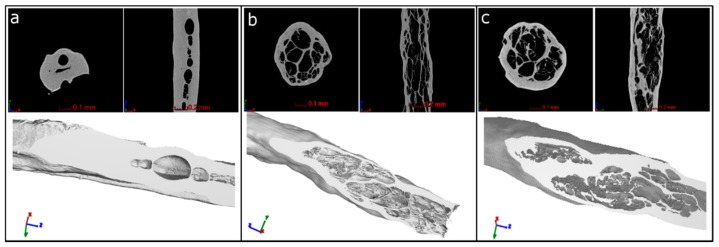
NanoCT images of a cross section and logitudinal section of a single strand of (a) PA; (b) AGG2; (c) AGG3. Bottom images: 3D reconstructed images of the CT scans with applied slice filter.
